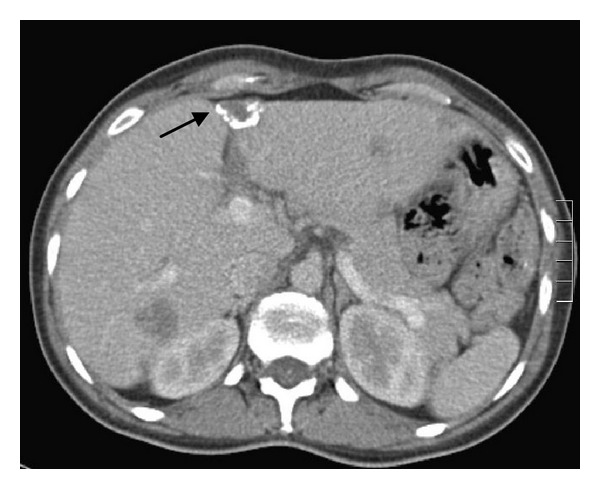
Abdominal CT scan, with intravenous contrast showing several hypodense lesions on both liver lobes. Arrow shows lesion with calcifications.
